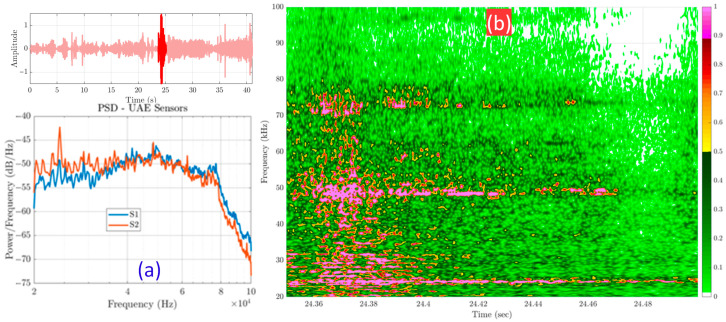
Detailed view of wayside noise. (a) A representative high-energy burst and its broadband PSD. (b) The corresponding spectrogram, showing a dense and pattern less “wall of sound” across the ultrasonic spectrum.
